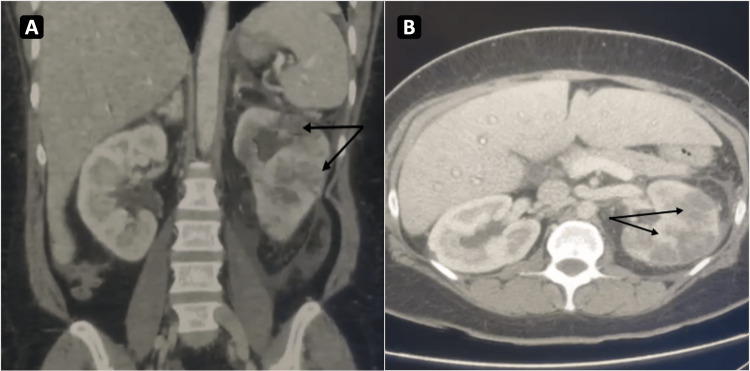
CT scan before intervention (A) Coronal sectional view of enhanced CT of the abdomen with contrast revealed a normal right kidney and a mildly enlarged left kidney with heterogeneous enhancement (black arrows) showing multiple wedge-like hypodense areas involving the renal cortex. (B) Cross-sectional view of the enhanced CT of the abdomen revealed signs of emphysematous changes (black arrows) and air pockets communicating with the adjacent pelvicalyceal system.
